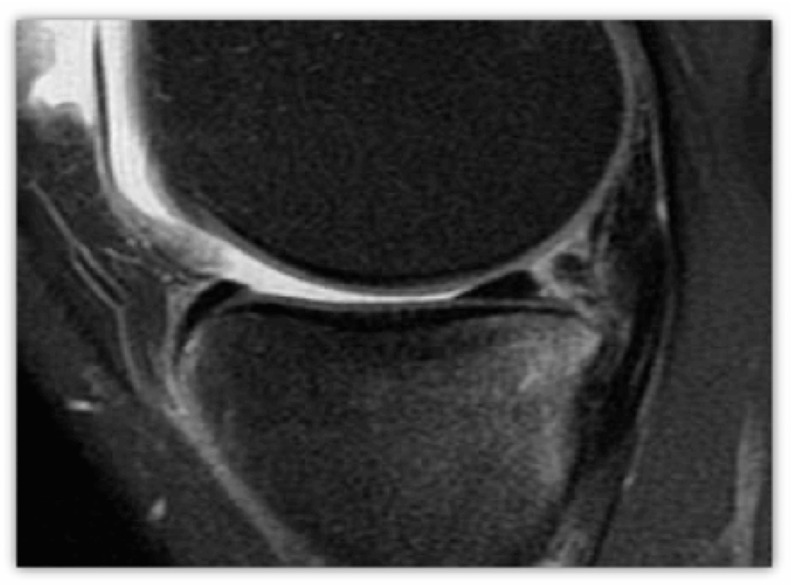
Example of a true positive, found to be repairable by all 3 reviewers (score = 4) and repaired intraoperatively. This sagittal proton density fat saturation MRI of the knee shows a longitudinal tear through the posterior horn of the medial meniscus, with a vertically oriented high-signal cleft at its posterior periphery and bone marrow edema within the posterior medial tibial plateau.
